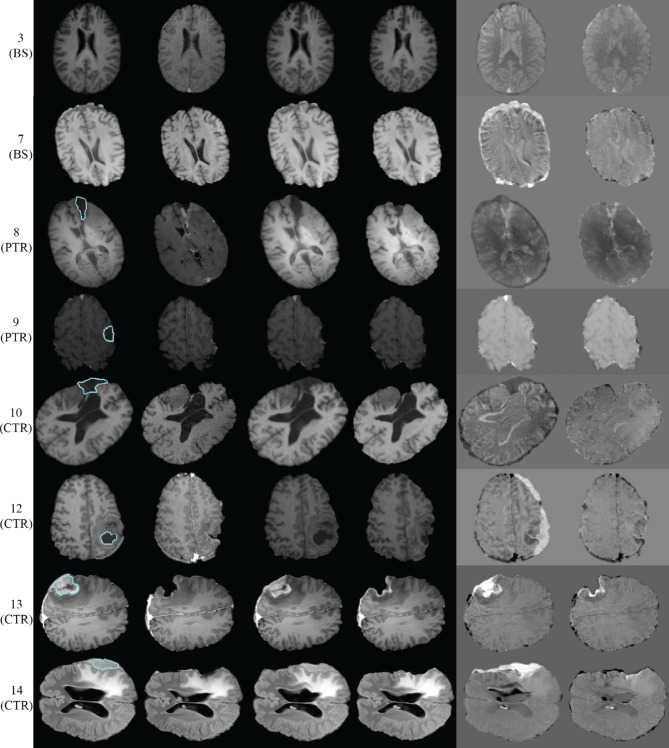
Qualitative evaluation results for 8 of the 14 case studies. Each row represents a single case. The left margin indicates the number and the type of each case. From left to right column: pre-op MRI, intra-op MRI, warped pre-op MRI (PBNRR), warped pre-op MRI (APBNRR), warped pre-op MRI (PBNRR) subtracted from intra-op MRI, warped pre-op MRI (APBNRR) subtracted from intra-op MRI. For the PTR and CTR cases, the cyan color delineates the tumor segmentation in the pre-op MRI. The BS cases do not require tumor segmentation.
